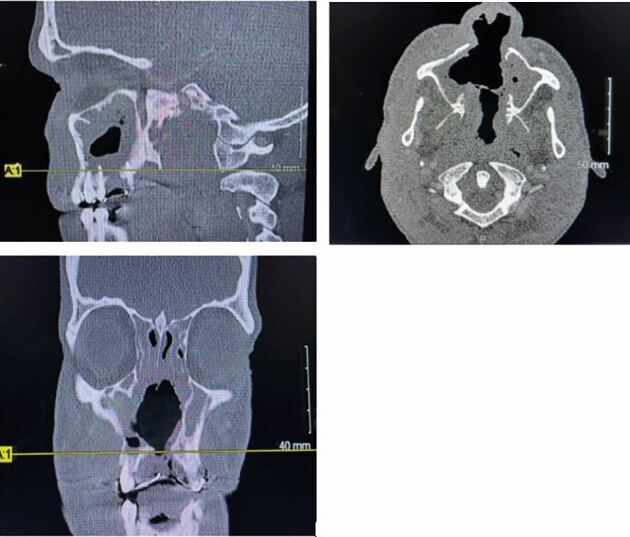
Head and neck CT for Case 1, highlighting the extent of the CIMDL
